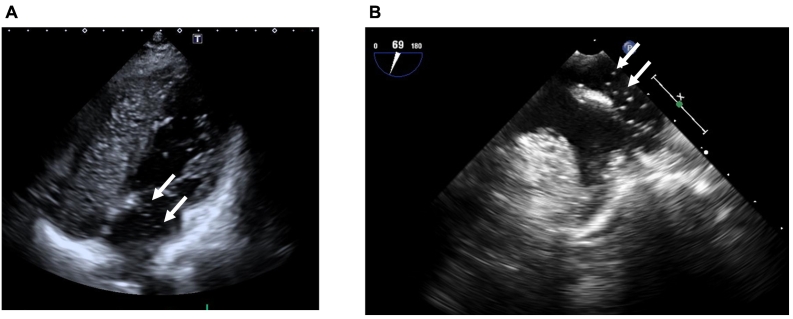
Saline contrast tests. (A) Microbubbles in the left atrium three cardiac cycles after detection in the right atrium on transthoracic echocardiography (arrows). (B) Microbubbles flowing into the left atrium through left upper pulmonary vein on transesophageal echocardiography (arrows).
